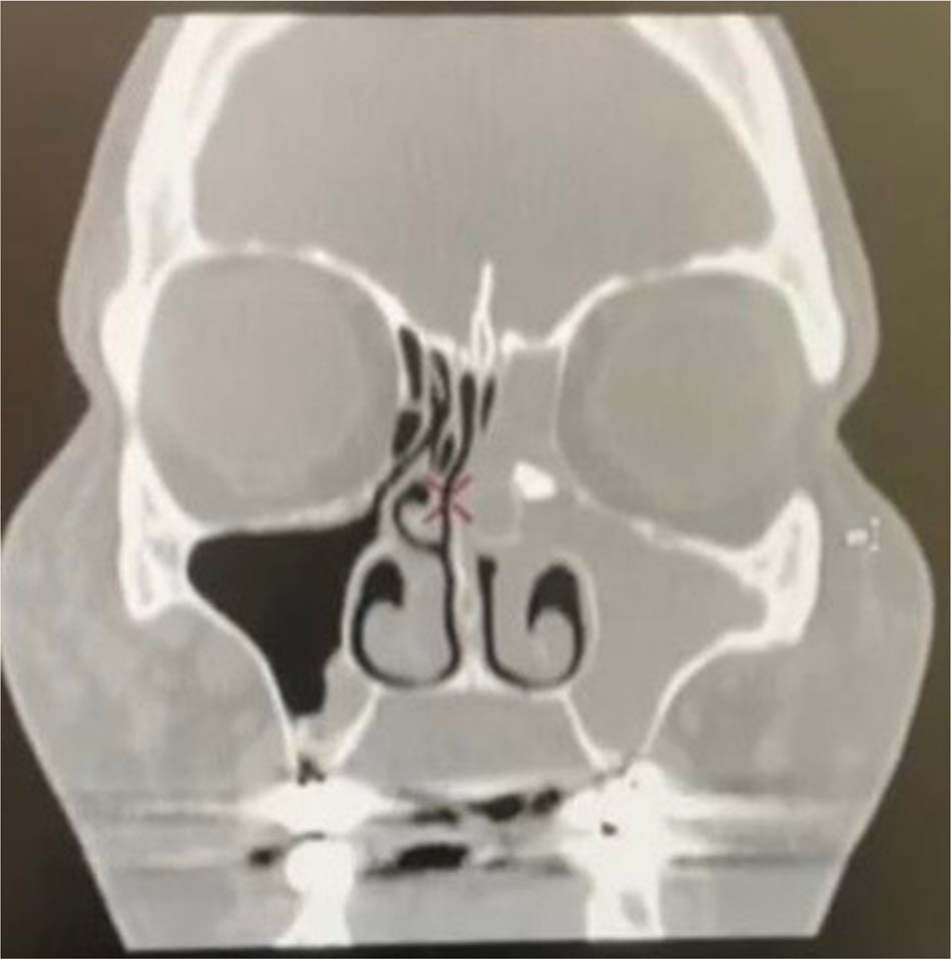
Coronal computed tomography image showing left maxillary and ethmoid sinusitis and a foreign body in the left anterior ethmoid sinus.
